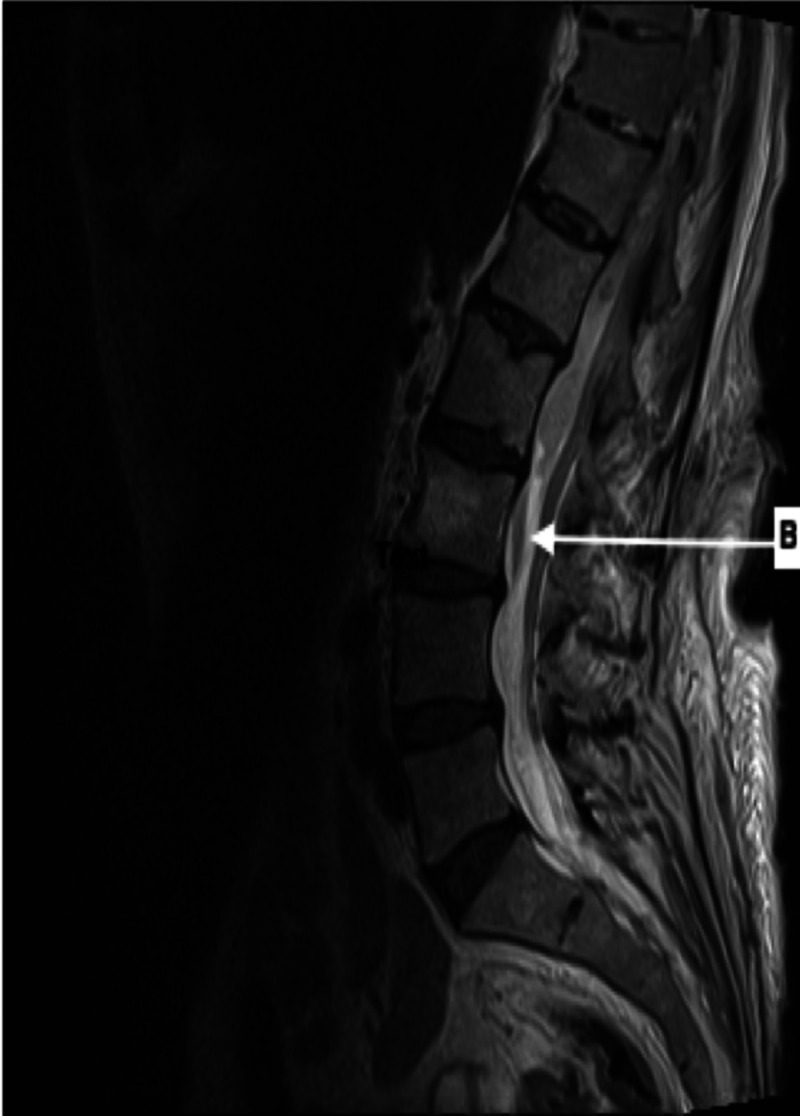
Magnetic resonance imaging T2 sagittal view Arrow B represents an additional area of heterogeneous collection posterior to L3 extending for 2.3 cm in length
